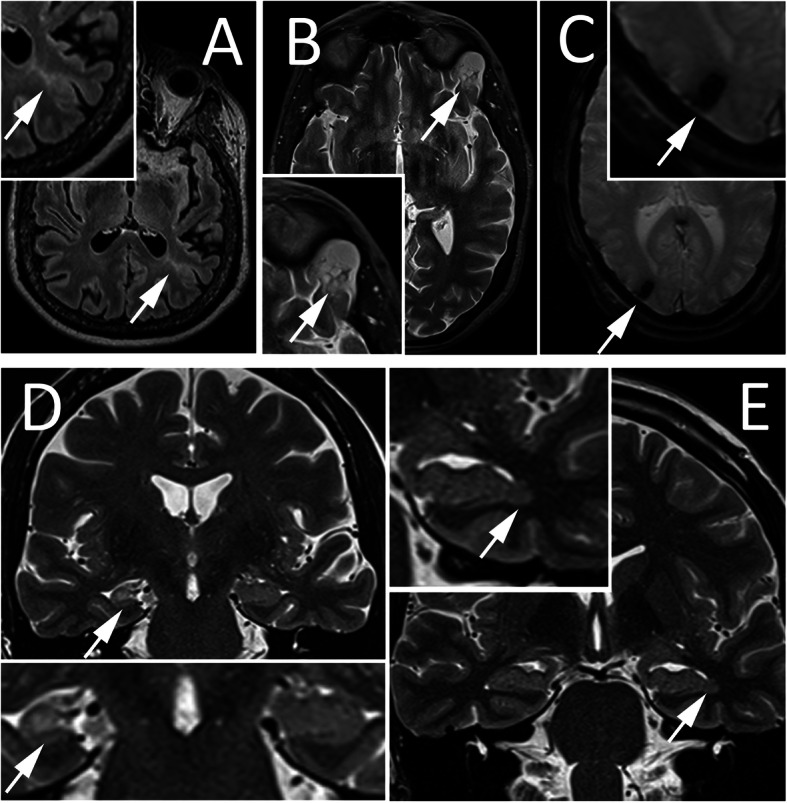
Representative images of epileptogenic lesions in MRI sets C. A Left frontal cortical dysplasia, axial FLAIR. B left frontal long-term epilepsy associated tumor, axial T2. C Right occipital hemosiderin deposits after hemorrhage, axial T2*. D right hippocampal sclerosis, coronal T2. E left periventricular nodular heterotopia, coronal T2
